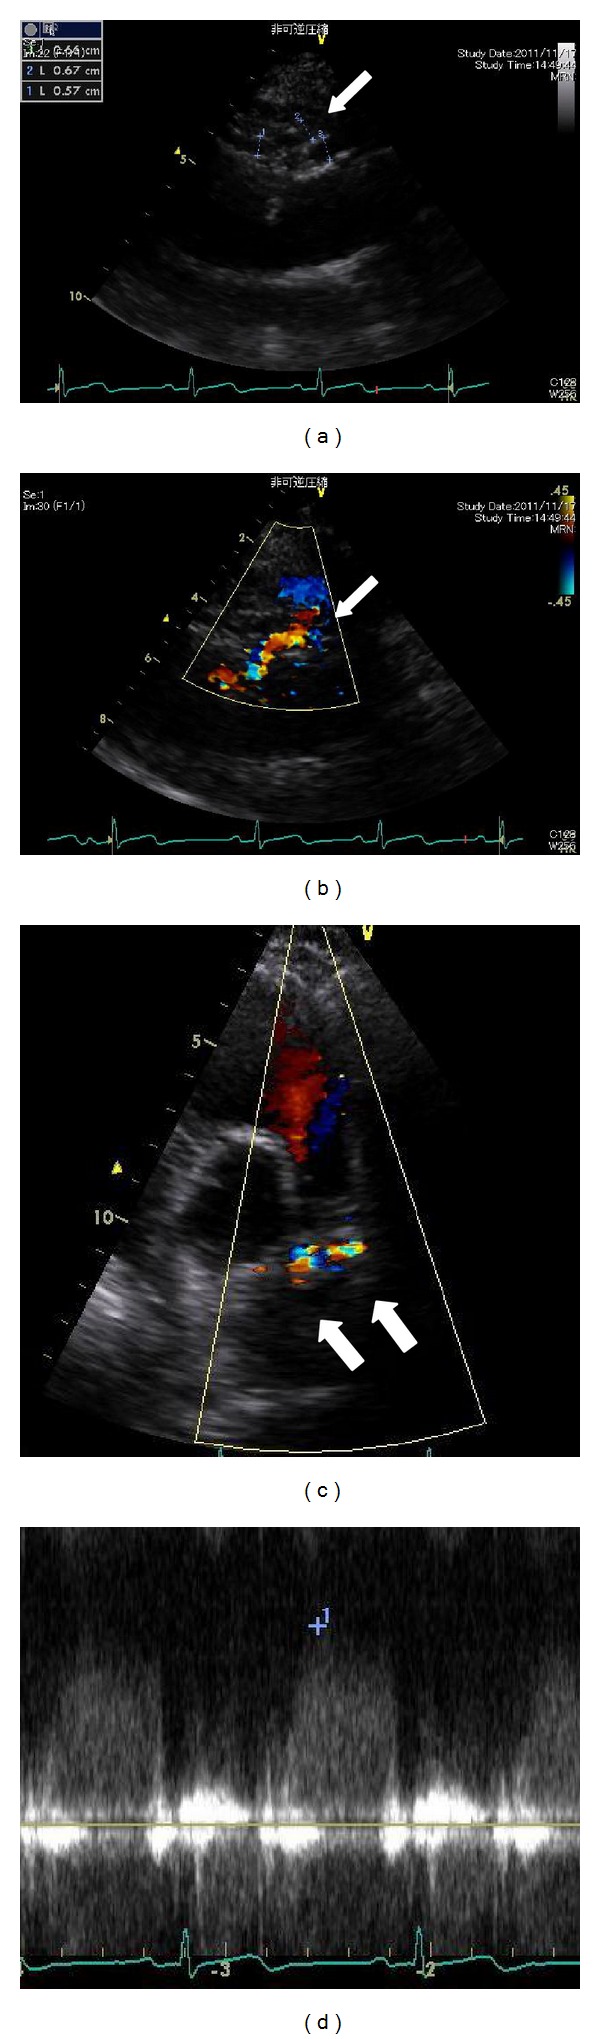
Transthoracic echocardiography. Abnormal, tortuous vessels were observed around the right coronary artery, and flow signals were detected by Doppler color flow imaging (a), (b). Abnormal vessels, predominantly exhibiting diastolic flow signals, were observed around the pulmonary trunk (c), (d).
